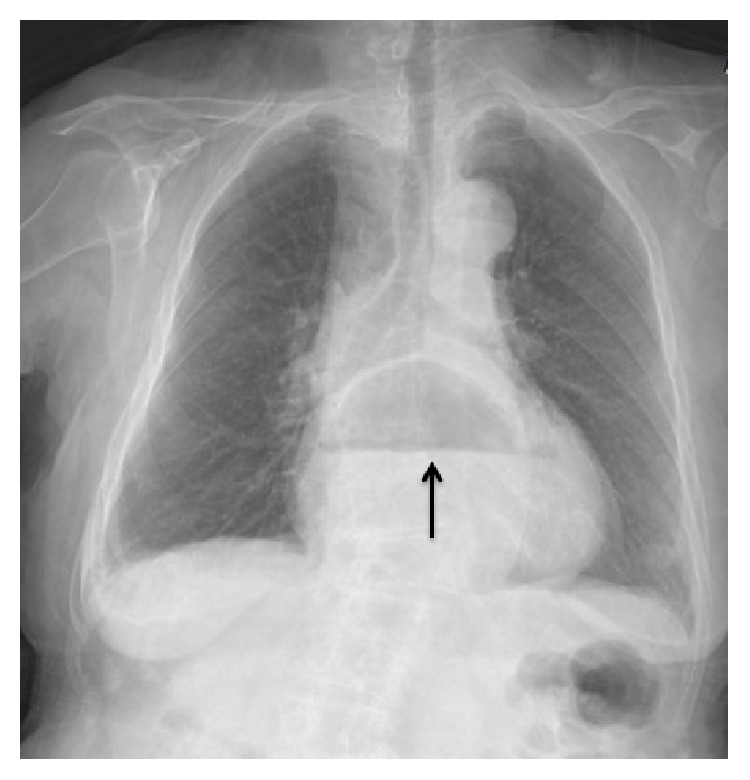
Appearance of air-fluid level superposed with cardiac contour on posterioanterior chest radiograph.
